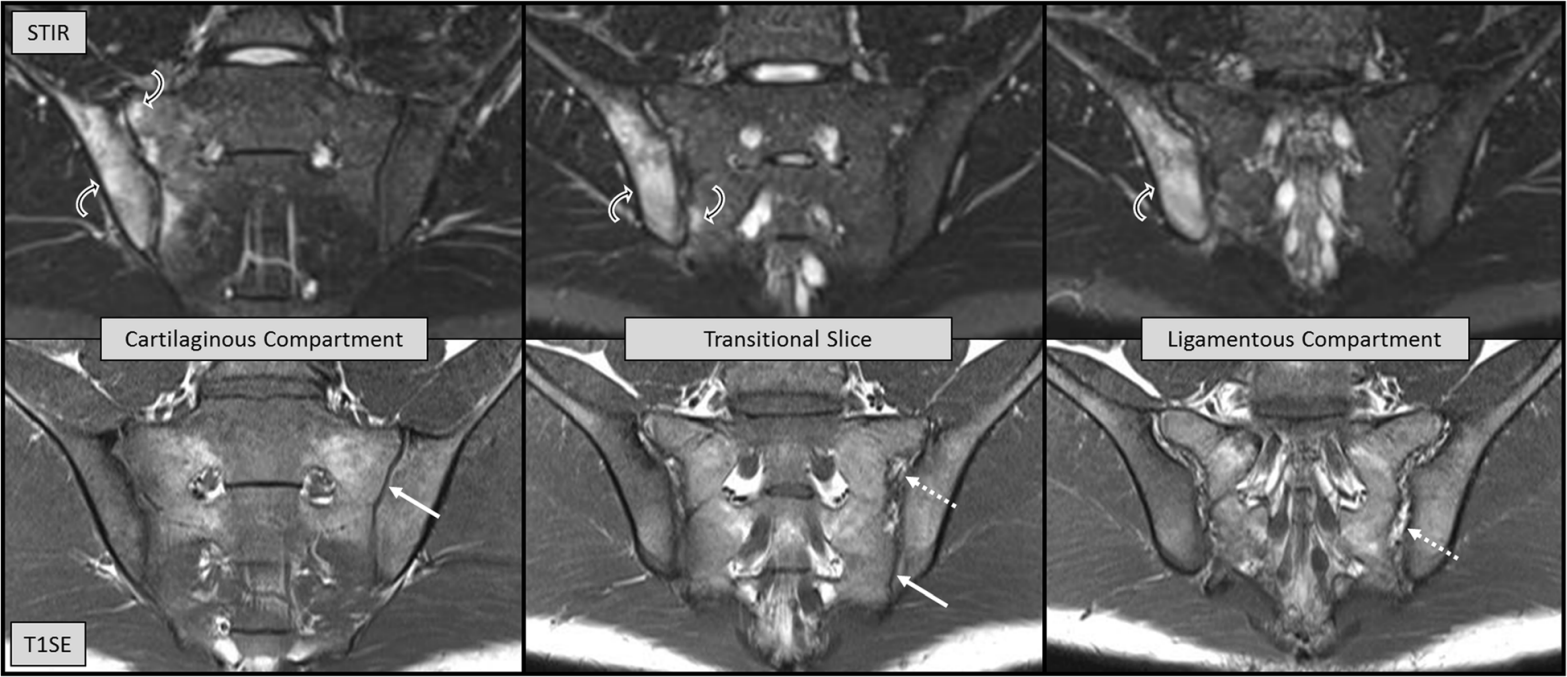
MRI studies of a 22-year-old man, human leucocyte antigen B27-positive, with a 4-month history of inflammatory back pain. The radiographic modified New York criteria were not met, and C-reactive protein was 7 mg/l (reference limit ≤5 mg/l). The upper and lower rows show short tau inversion recovery (STIR) and corresponding T1 spin echo (T1SE) sequences, respectively. The left panel represents an MRI slice from the cartilaginous compartment (*solid white arrow* on T1SE), the right panel from the ligamentous compartment (*broken white arrow* on T1SE), and the central panel is a transitional slice containing parts of both the cartilaginous and ligamentous compartment (*solid and broken white arrows* on T1SE). The black and white pattern of the space between the ilium and the sacrum in the ligamentous compartment has its origin on the T1SE images in sacroiliac ligaments which have low MRI signal intensity surrounded by fat tissue which is bright on T1SE. On the STIR sequence, the ligamentous compartment is comprised of low signal ligaments and fat tissue as the fat signal is suppressed on STIR, and the bright signal relates to fluid in the lumen of blood vessels. In this patient with nonradiographic axial spondyloarthritis, bone marrow edema (*curved white and black arrows* on STIR) predominantly in the iliac part of the right sacroiliac joint extends from the cartilaginous into the ligamentous compartment
